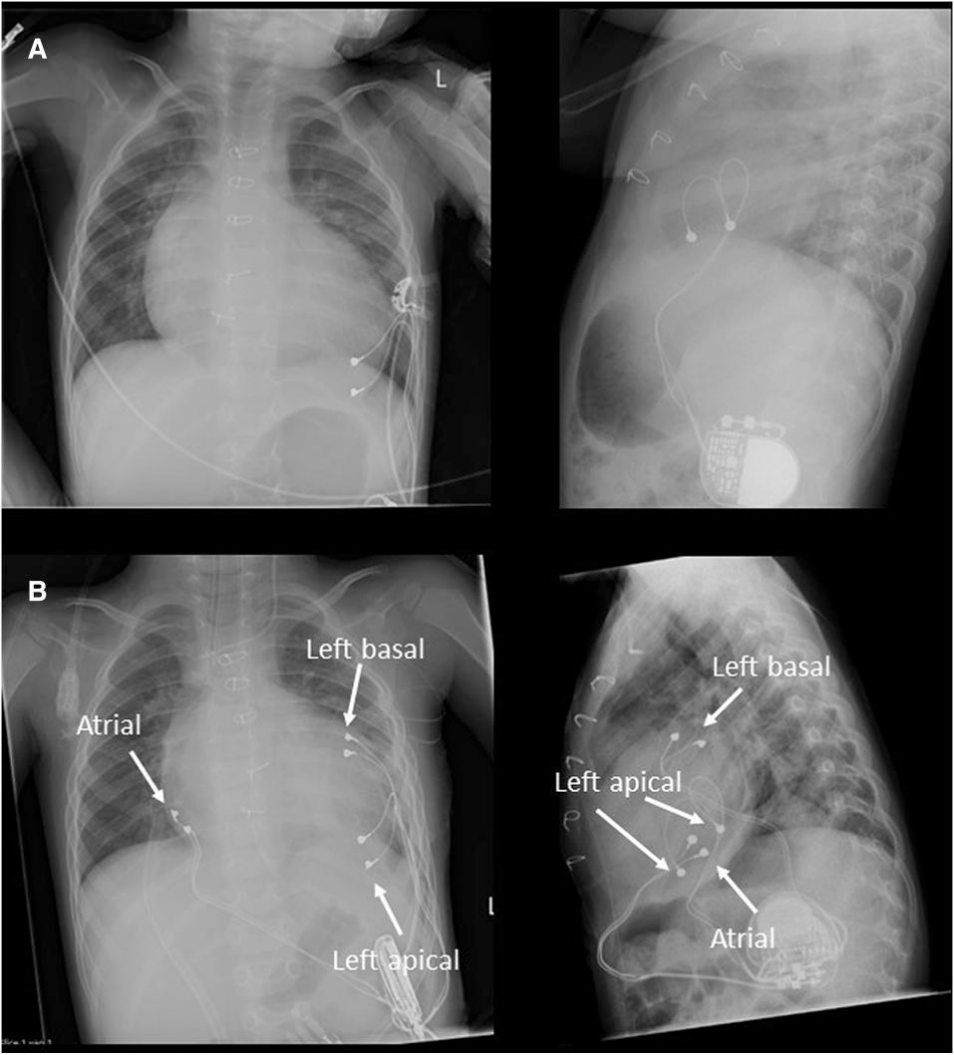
(*A*) Anteroposterior and lateral chest radiograph with apical left ventricular (LV) pacemaker leads after initial pacemaker insertion. (*B*) Anteroposterior and lateral chest radiograph with the original LV leads, additional LV basal free wall, and atrial leads.
